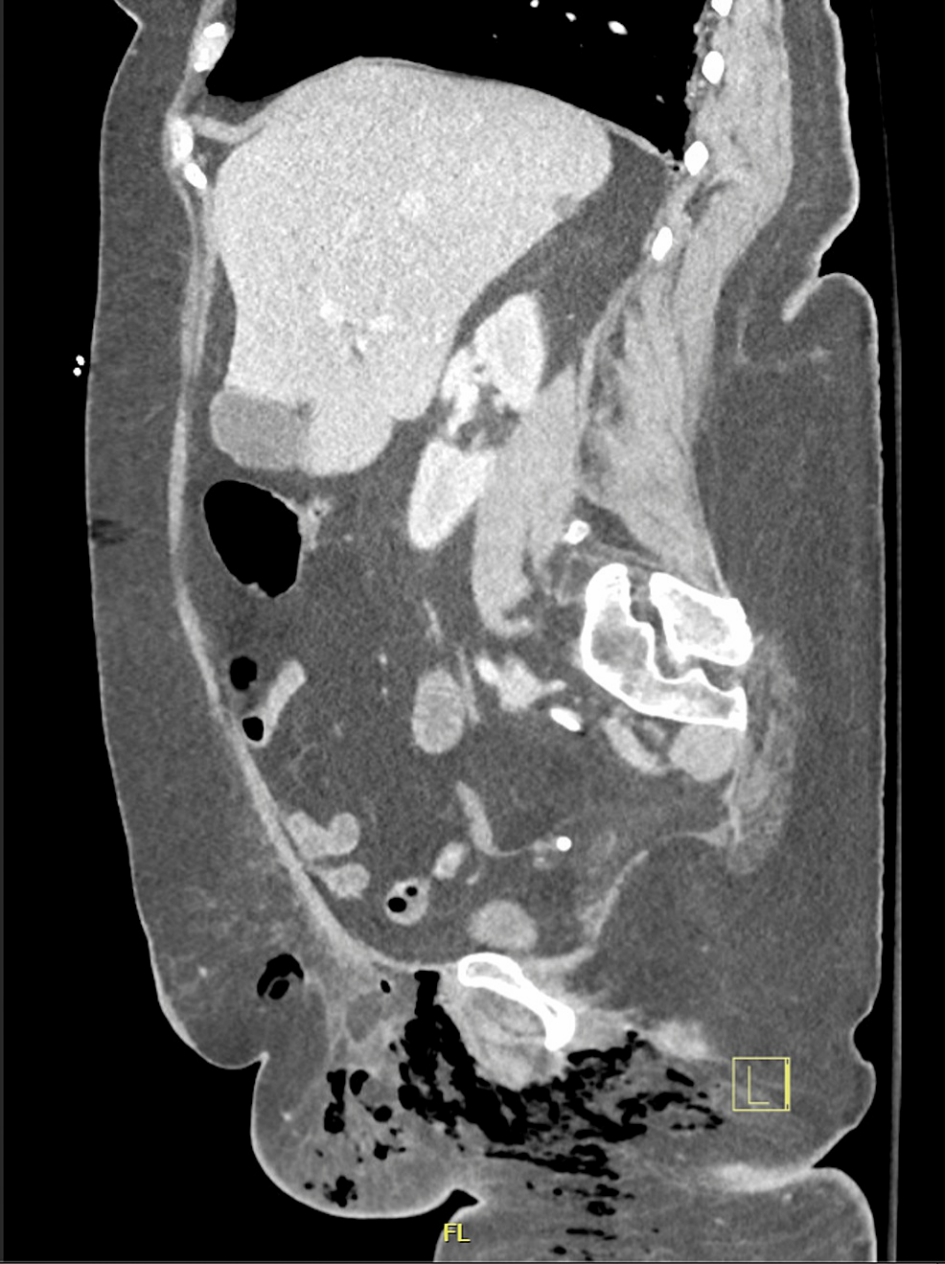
CT (sagittal view) with contrast of the abdomen and pelvis Subcutaneous gas was visible in the right labia, right adductors, and perineum.
